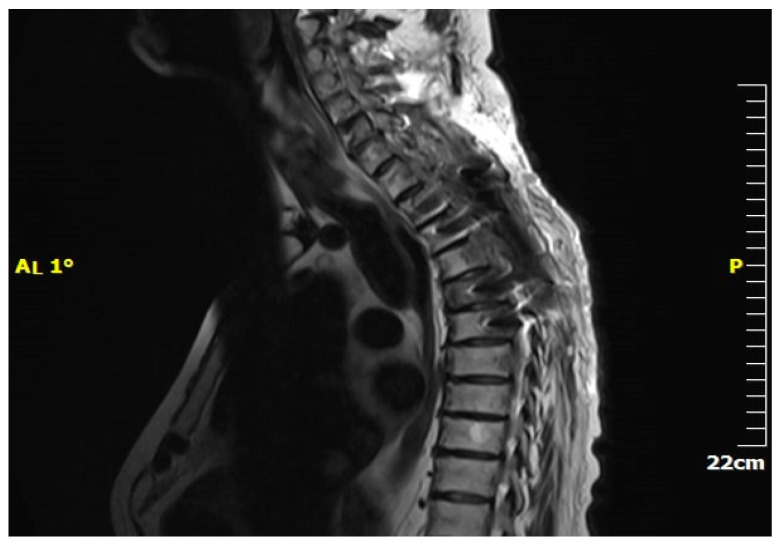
Post-surgical MRI—thoracic spinal fusion following mechanical instability caused by prostatic tumour metastasis.
